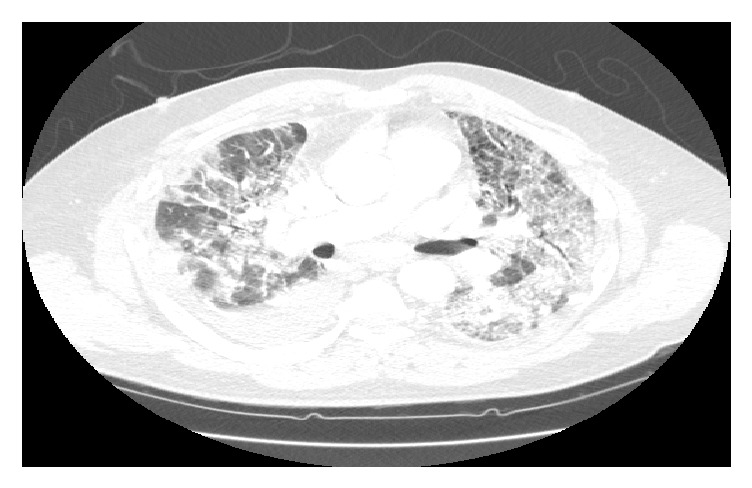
Chest CT angiogram taken during the acute exacerbation. Right pleural fluid filling 1/4 of chest, bilateral interstitial, and airspace disease. Ground glass opacities, pathological adenopathies in the mediastinum, cardiomegaly, and no pulmonary embolus.
